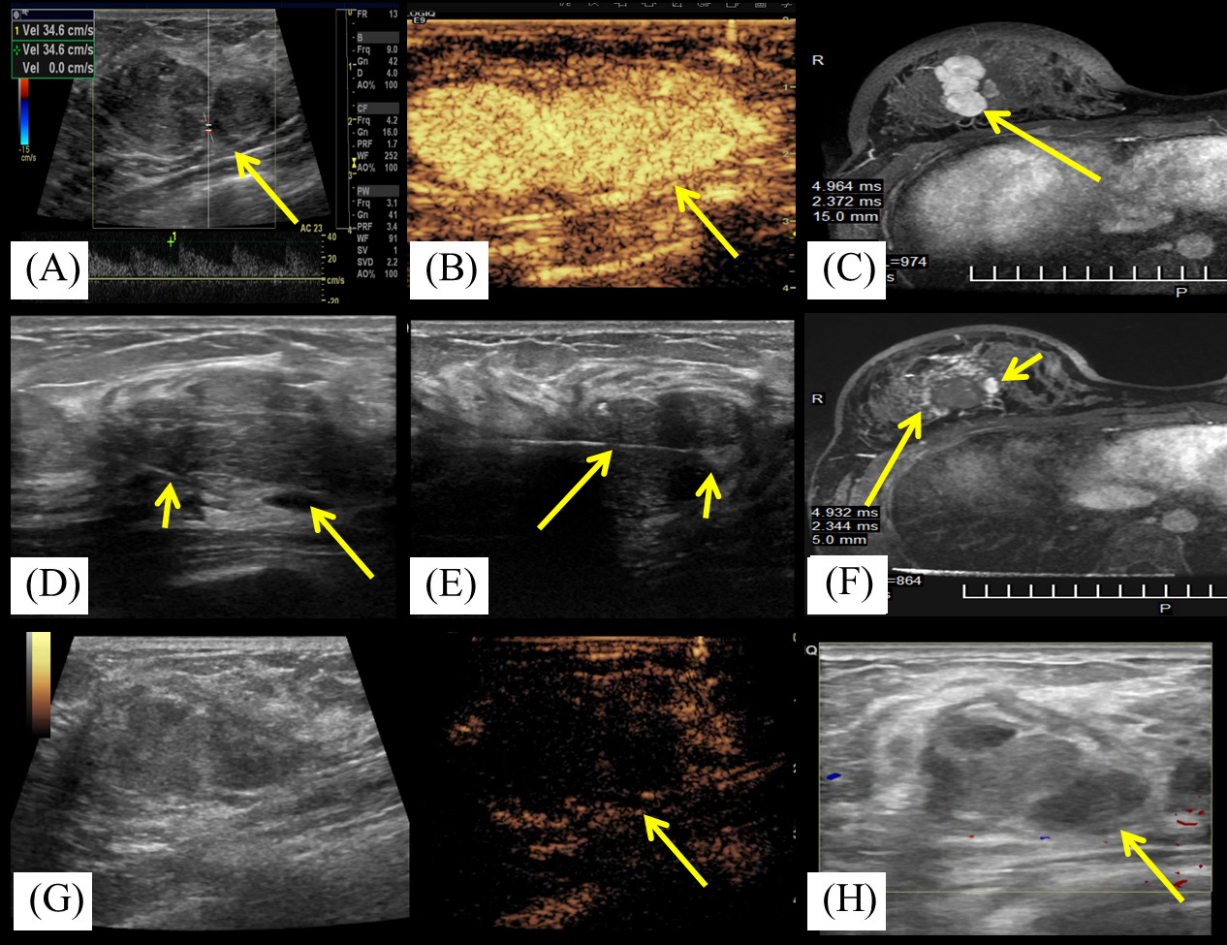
A 19-year-old woman with right breast fibroadenoma A. US scan before MWA shows the hypoechoic mass with arterial blood flow signal with the rate of 34.6cm/s. The mass is adjacent to the pectoralis and the size is 3.6cmx2.4cmx1.7cm. B. Contrast-enhanced US before MWA shows the mass is hyper-enhancement (arrow) in arterial phase. C. Transverse contrast-enhanced MRI shows hyperintensity mass in right breast (arrow) before MWA in arterial phase. D. US scan before MWA shows the fluid (arrow) infused by the fine needle (small arrow) between the mass and the pectoralis. E. US scan shows the centrally placed antenna (arrow) in the mass and increased echogenicity near the irradiating segment of the antenna (small arrow) at the beginning of MWA session. F. Contrast-enhanced MRI image shows hypointensity treatment zone (arrow) and the peripheral nodular enhancement (small arrow) in arterial phase at third day after MWA. G. Contrast enhanced US at second day after MWA shows the mass is non-enhancement (arrow) in arterial phase. H. US obtained 3 months after MWA shows the heterogeneously hypoechoic ablation zone without blood flow signal and with the size of 3.3cmx2.2cmx1.5cm.
